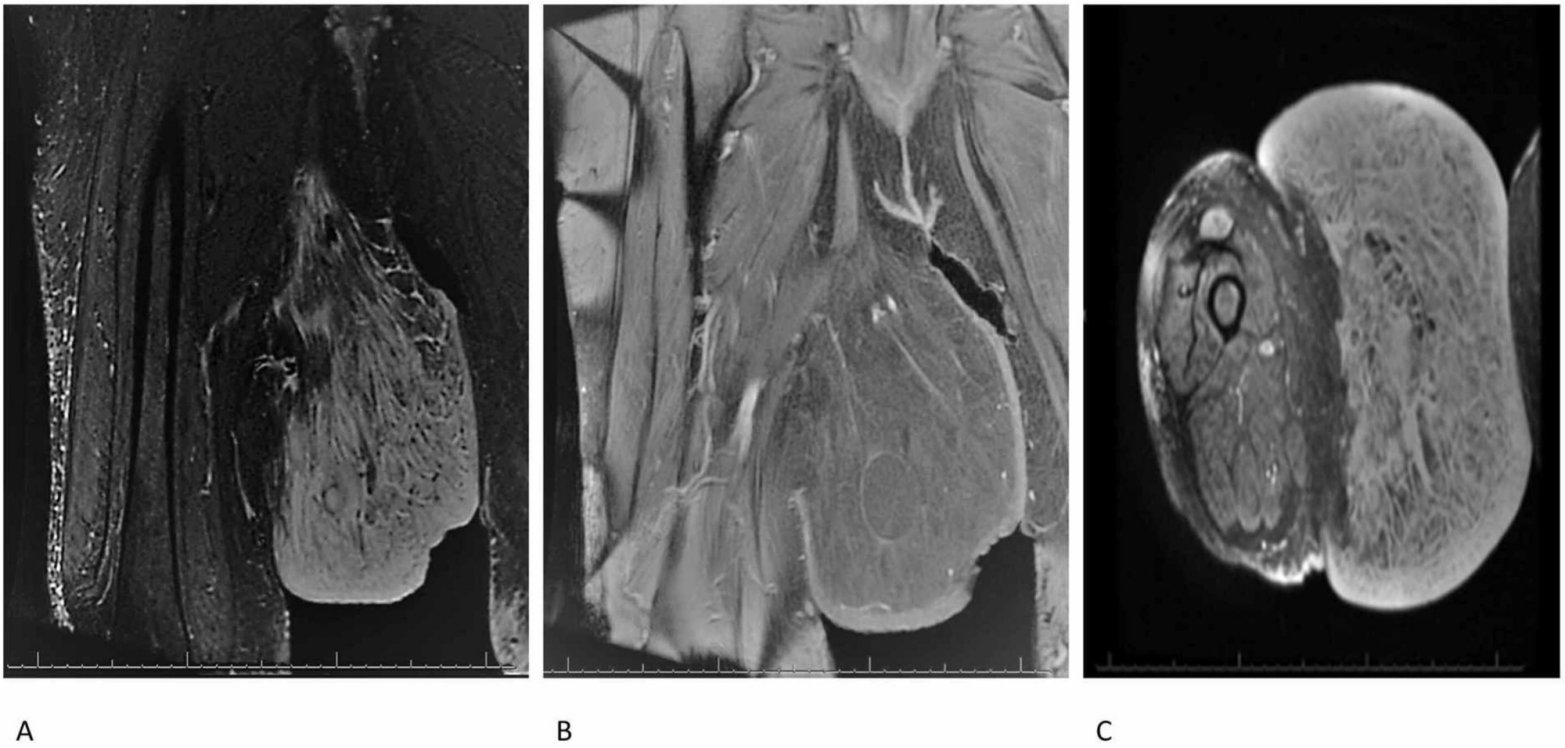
Preoperative magnetic resonance images of the lesion A and B: coronal plane; C: axial plane
